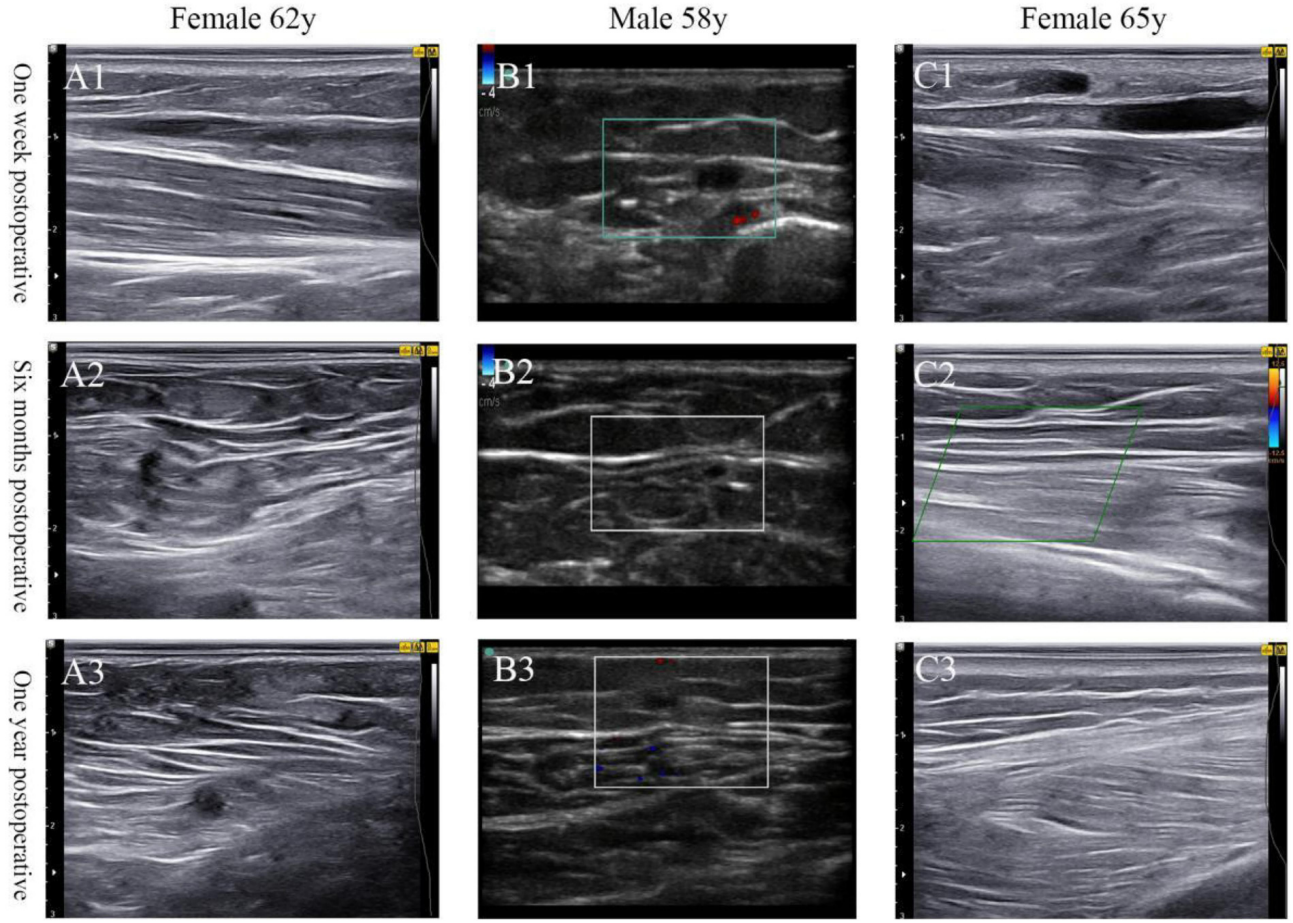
Follow-up ultrasound results of three patient post-surgery. (A1,B1,C1) One week postoperative, (A2,B2,C2) six months postoperative, (A3,B3,C3) one year postoperative.
